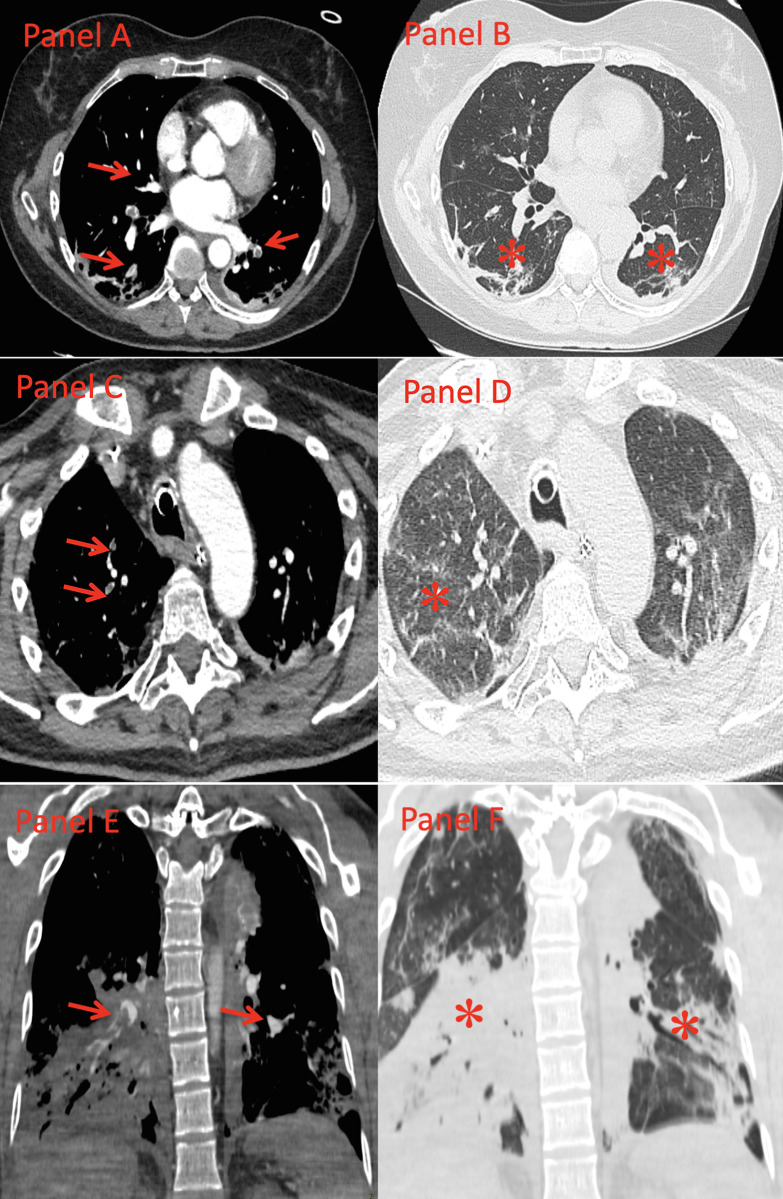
Localization of pulmonary embolism and pneumonia. (A,B) Computed Tomography Pulmonary Angiography shows intraluminal filling defects in some segmental pulmonary arteries of both lower lobes (Panel A, red arrows), localized in lung areas of normal attenuation (Panel B). Subpleural band atelectasis are evident in both lower lobes (Panel B, asterisks). SCORE 0. (C,D) Computed Tomography Pulmonary Angiography shows intraluminal filling defects in two subsegmental pulmonary arteries of the right upper lobe (Panel C, arrows), localized in areas of ground glass attenuation (Panel D, asterisks). A further intraluminal filling defect, not displayed, is present in a segmental pulmonary artery of the right lower lobe. SCORE 2. (E,F) Computed Tomography Pulmonary Angiography shows intraluminal filling defects in some segmental pulmonary arteries of both lower lobes (Panel E, arrows), all localized in areas of lung consolidation (Panel F, asterisks). SCORE 3.
